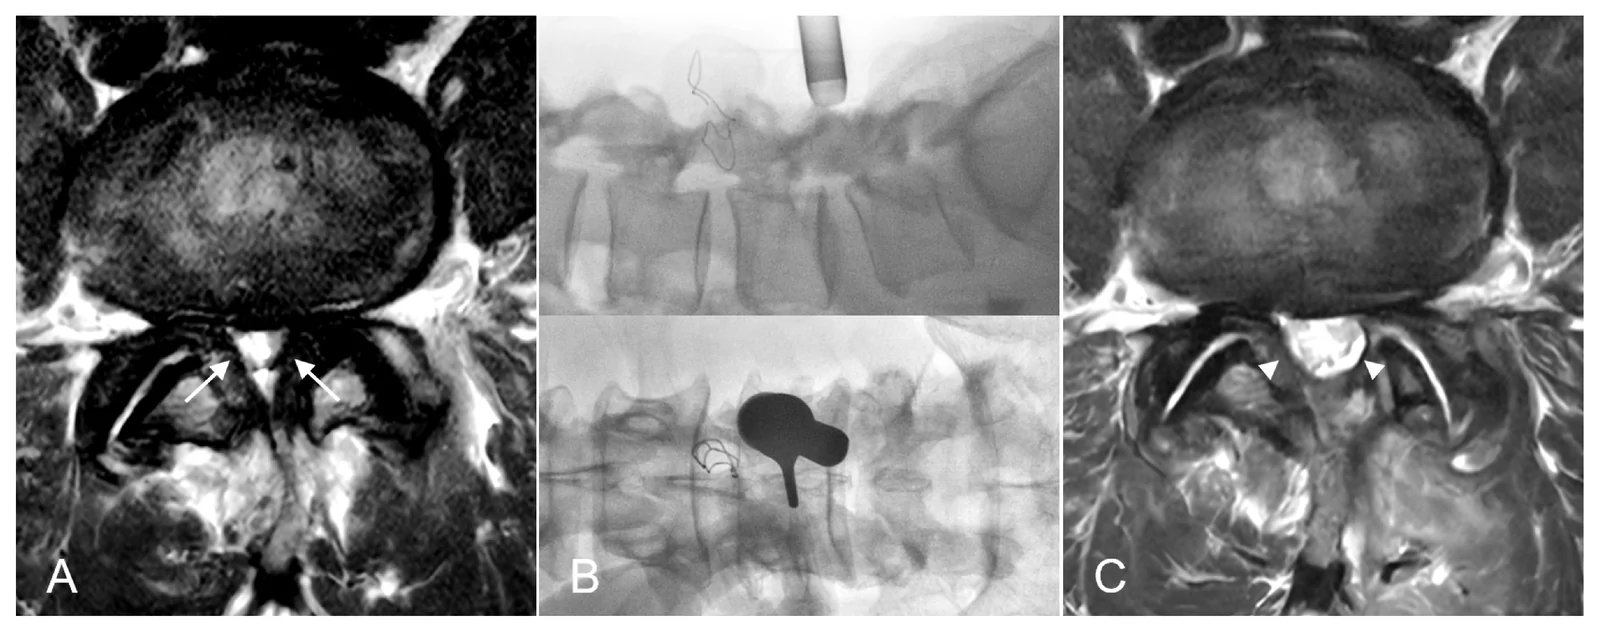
Representative case of a 78-year-old man with neurogenic intermittent claudication and bilateral radicular pain who underwent FE-ULBD under surgeon-directed local anesthesia. (A) Preoperative axial T2-weighted MRI demonstrating severe central lumbar spinal stenosis at the L4–5 level caused by hypertrophic ligamentum flavum and facet hypertrophy (arrows). (B) Intraoperative lateral (upper) and anteroposterior (lower) fluoroscopic images showing correct placement of the endoscopic working sheath at the L4–5 interlaminar space during FE-ULBD. (C) Postoperative axial T2-weighted MRI demonstrating successful over-the-top bilateral decompression with expansion of the dural sac and bilateral lateral recesses (arrowheads).
